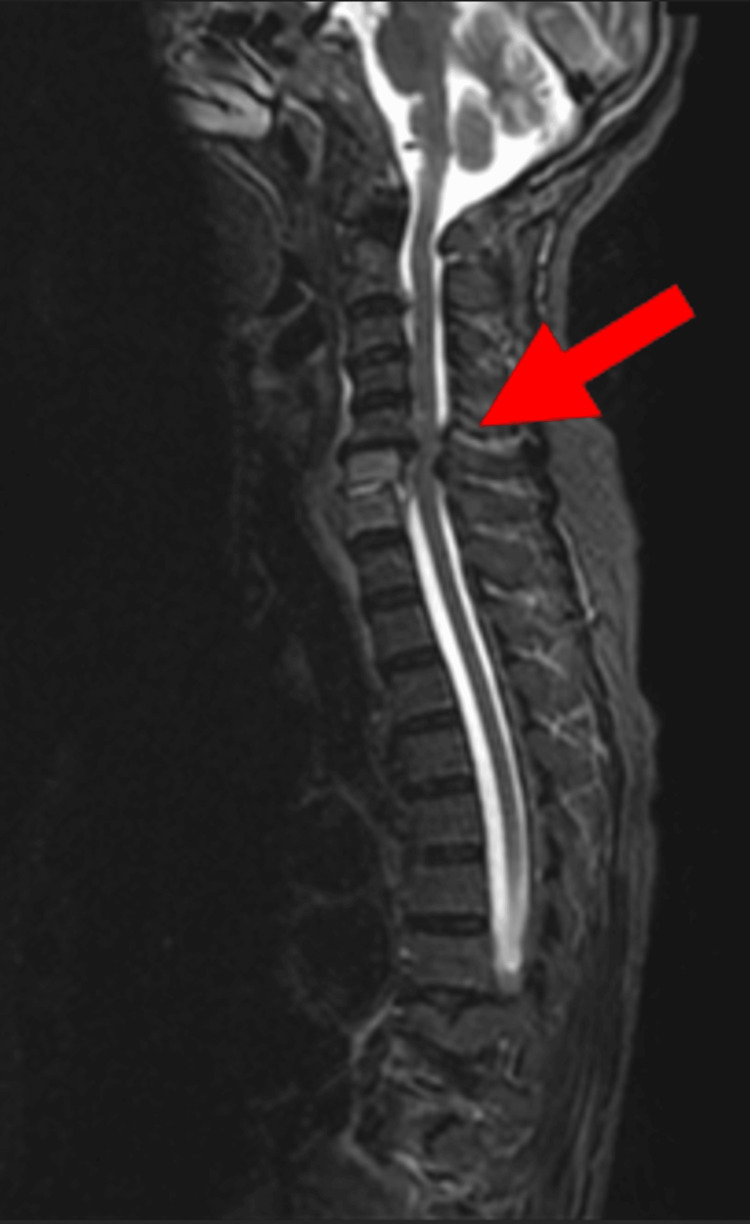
MRI findings of the entire spine. Short inversion time inversion recovery revealing a new high intensity at the L1 and L2 vertebral bodies (arrow).
